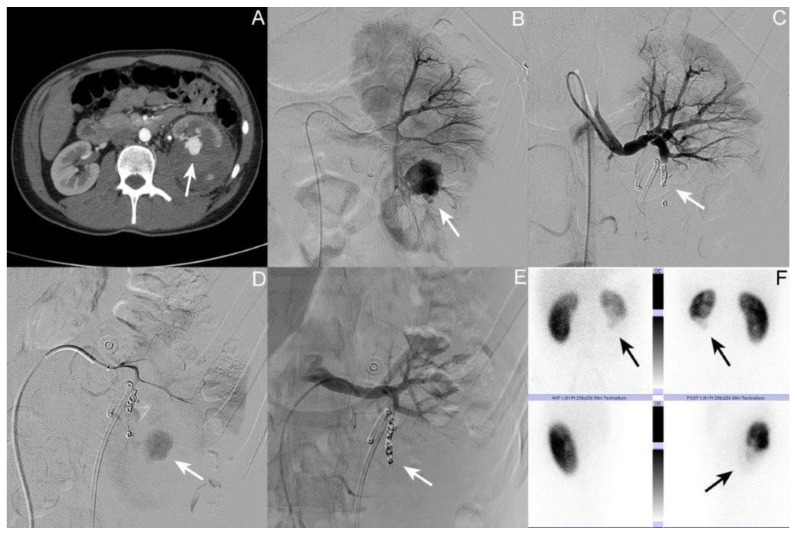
Selected imaging of case I. (A) Axial contrast-enhanced computed tomography (CT) scan of the abdomen; the arrow shows the 24 mm arteriovenous (AV) fistula at the lower pole of the left kidney. (B) Angiography of the left kidney; the arrow shows the AV fistula. (C) Angiography post coil embolization; the arrow shows the coils in situ in the lower pole segmental artery of the left kidney. (D) Repeat angiography showing persistent AV fistula (arrow). (E) Angiography post second coil embolization; the arrow shows the coils in situ in the lower pole segmental artery of the left kidney. (F) Technetium-99 m dimercaptosuccinic acid (DMSA) renal scintigraphy showing no tracer accumulation in the lower kidney pole on the left side (arrow); contribution right/left kidney 65%:35%.
